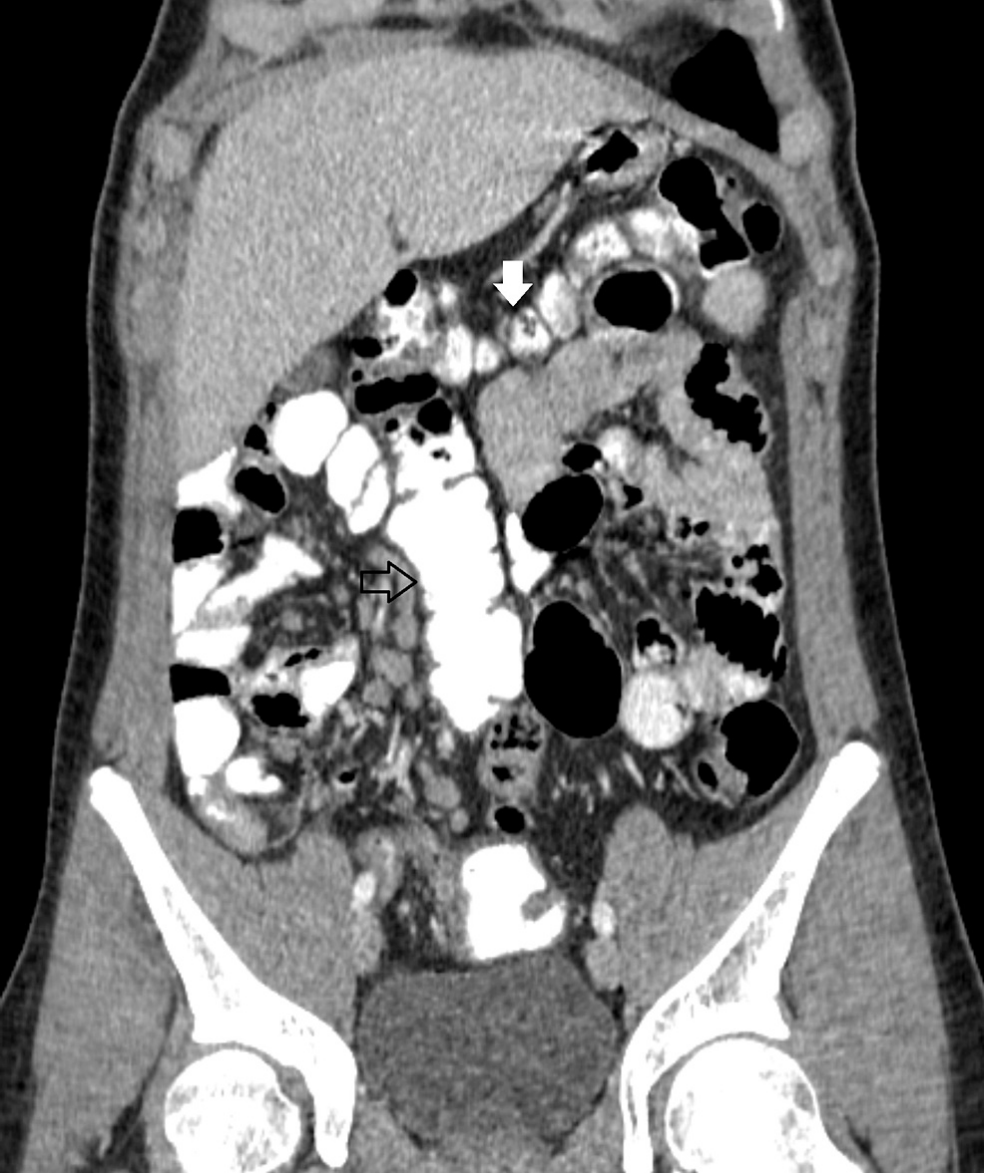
Enhanced CT with oral contrast showing the transverse colon (white arrow) lying lateral to the ascending (blue arrow) towards the left abdomen
